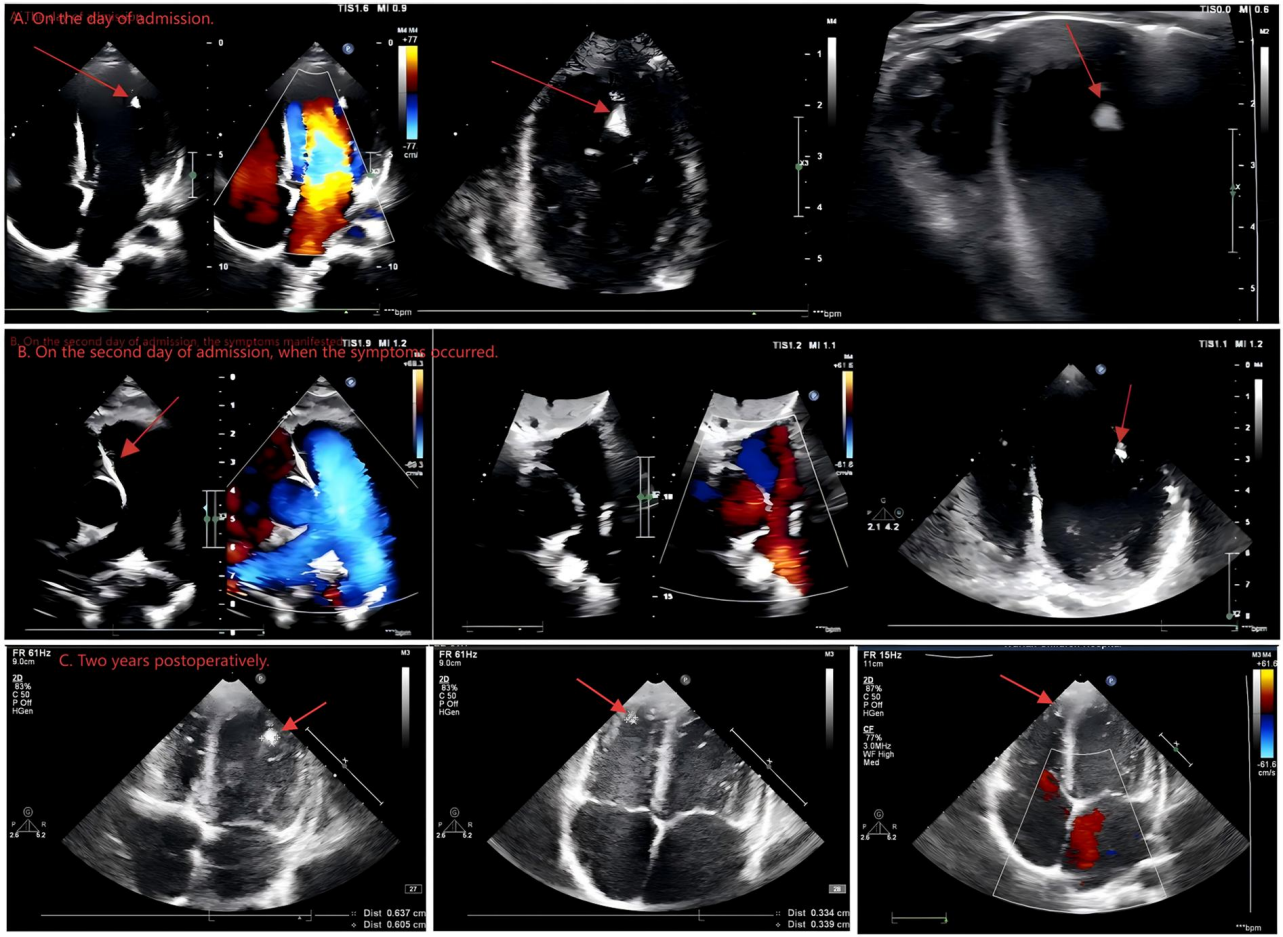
Cardiac ultrasound examination. (A) On the day of admission. (B) On the second day of admission, when the symptoms occurred. (C) Two years postoperatively.
